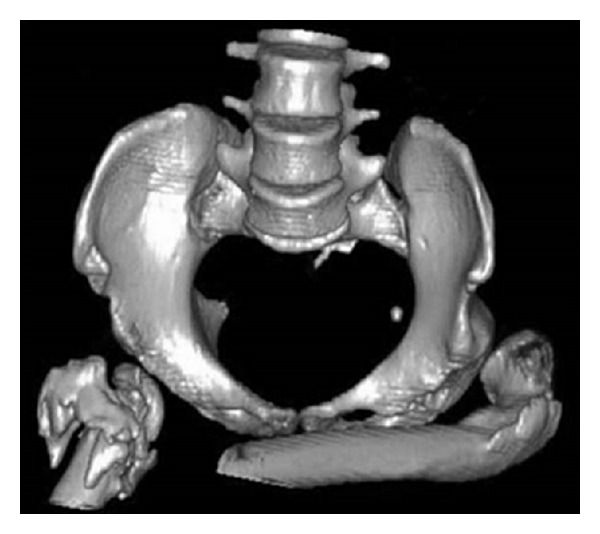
Case  4  preoperative CT.
